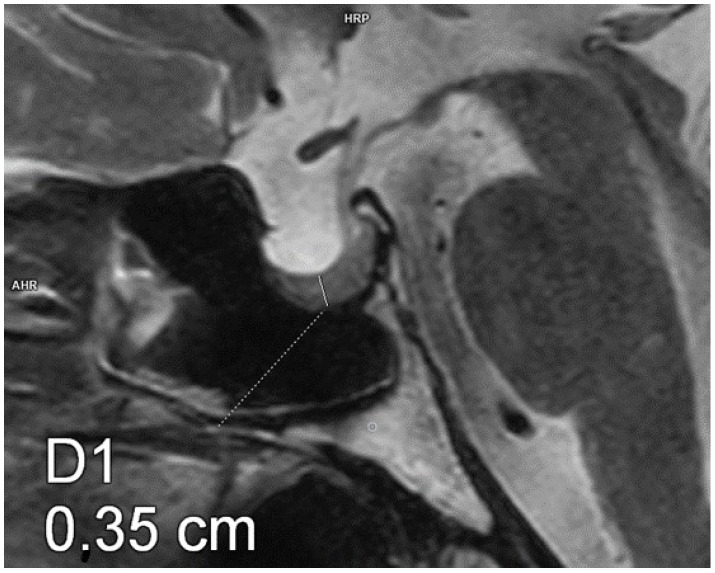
Partial empty sella. Magnetic resonance imaging, T2-weighted image, sagittal plane. The craniocaudal diameter of the pituitary gland is 3.5 mm.
